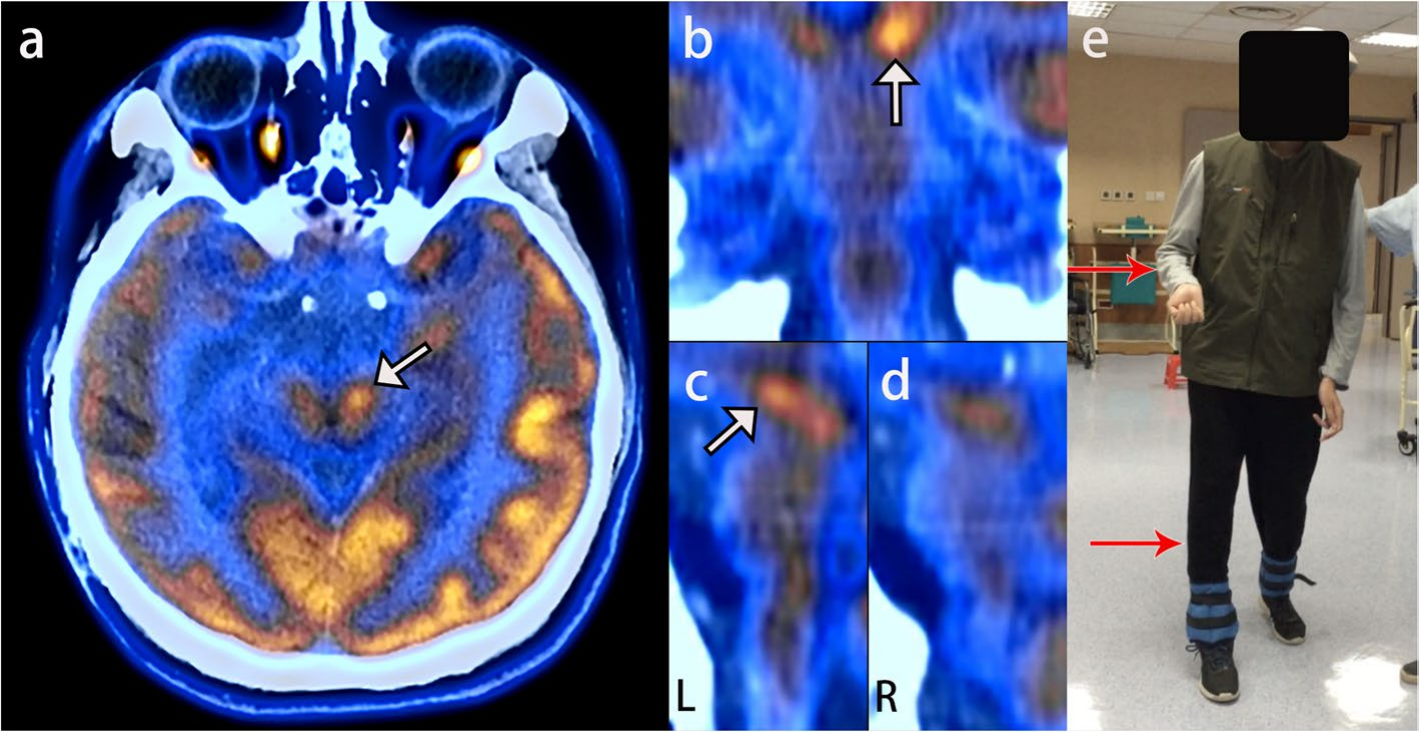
Patient was food-deprived 6 h before positron emission tomography/computed tomography (PET/CT) scanning. Emission data were acquired for 50 min after an intravenous bolus injection of 292 MBq fluorine-18 fluorodeoxyglucose (18 F-FDG). The mean standardized uptake value of each cerebral peduncle was measured on fused PET and CT image. PET/CT imaging demonstrated a focal region of intense 18 F-FDG uptake in the left cerebral peduncle (white arrow). a Axial view. b Coronal view. c Left parasagittal view. d In the right parasagittal view of PET/CT imaging at the level of the brainstem, no intense 18 F-FDG uptake can be seen above the pyramidal decussation. e The patient had right-sided muscular rigidity (red arrows) and was able to walk unassisted after rehabilitation treatment
